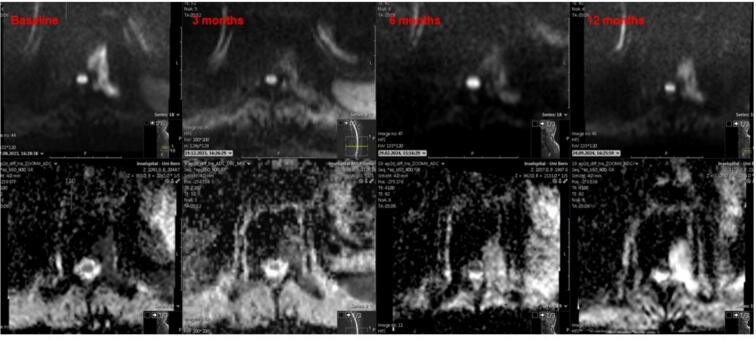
Baseline and serial DWI (upper row)/ADC (lower row) MRI images obtained at 3, 6, and 12 months following SBRT to a T10 metastasis (same patient in figure 3).
